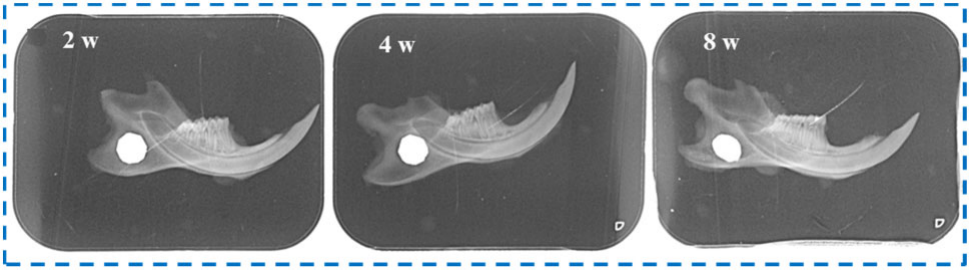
X-ray observation of mandible defect implanted with Zn0.8Mn0.1Li scaffold.
